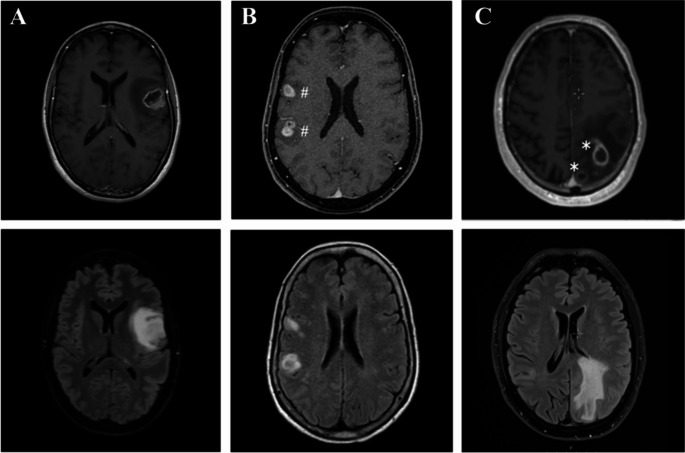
MRI T1 with Gadolinium contrast enhancement (upper) and T2 FLAIR (lower). Solitary lesion glioblastoma (A), multicentric glioblastoma without FLAIR interconnection (B) and multifocal glioblastoma (C)
